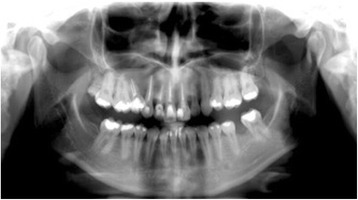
Panoramic radiograph showing a radiolucent lesion associated with teeth 13 and 15
